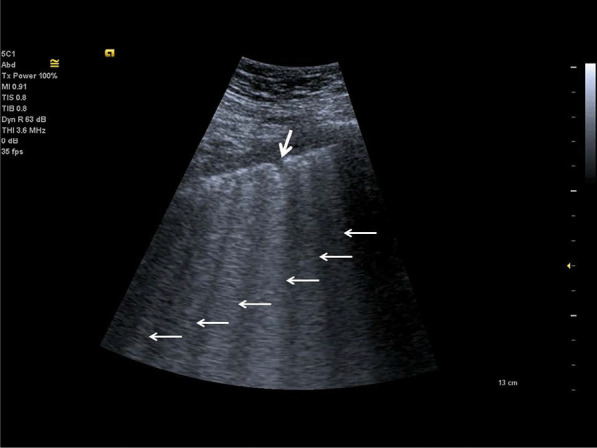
Lung ultrasonography, in comparison to Fig. 3, there is an increased density of B-lines (white arrows) indicating an interstitial syndrome of the lung with irregular, discontinuous pleural line with a small subpleural consolidation (bold white arrow)
